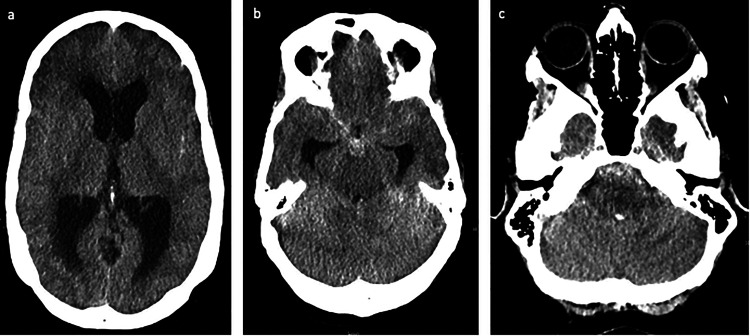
A 54-year-old female (patient 2) presenting with sudden onset of headache and altered mental status. Axial view of the noncontrast head CT showing (a) obstructive hydrocephalus, (b) SAH in the interpeduncular cistern and bilateral sylvian cisterns, and (c) SAH in the prepontine cistern and fourth ventricular IVH. CT: computed tomography; IVH: intraventricular hemorrhage; SAH: subarachnoid hemorrhage.
